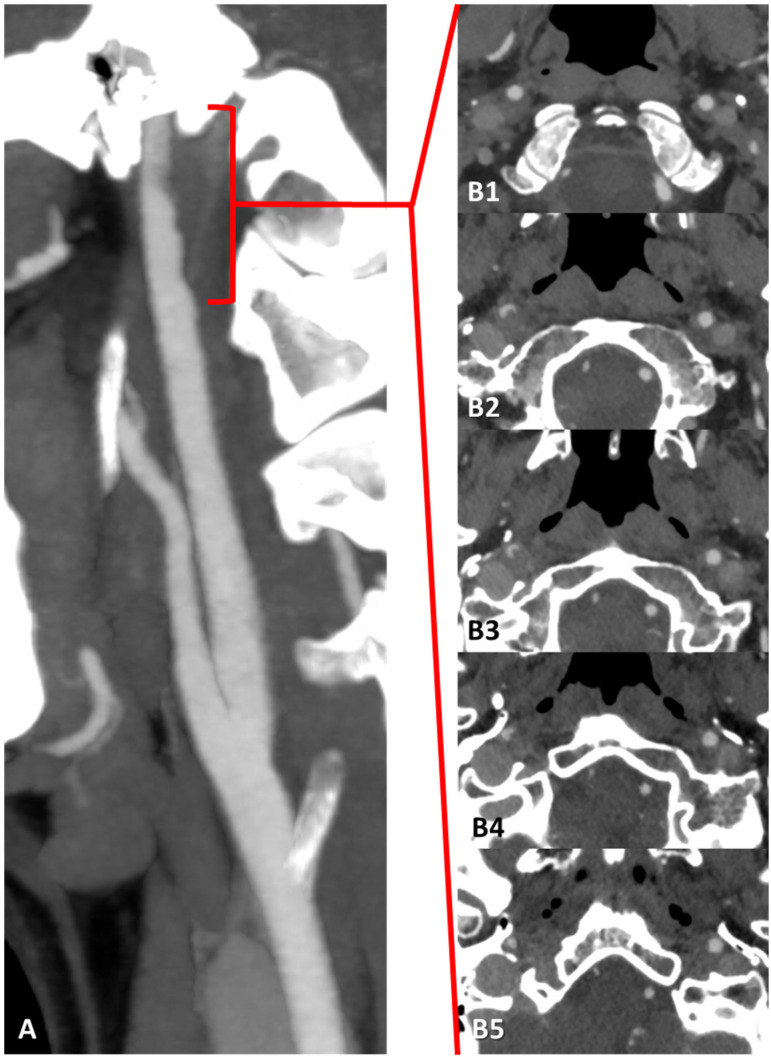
CTA in MIP/MPR reconstruction of the right ICA in oblique plane, showing an irregular luminal profile in the posterior wall in the prepetrous segment (A) (red square brackets). (B1–B5) are the corresponding source axial slices in a proximal-to-distal sequence, showing with better detail the hypodense structure protruding into the right ICA lumen as complicated atheroma with superimposed thrombus.
